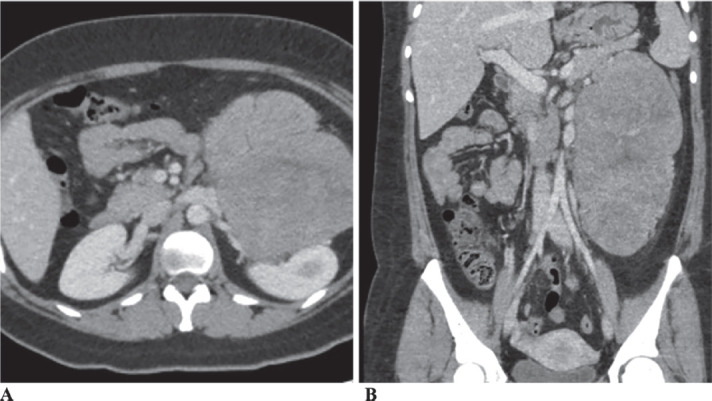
CT Abdomen (1A-Axial section and 1B-Coronal section) showing a 21 × 15 cm mass abutting the left kidney, left renal vessels, distal pancreas and spleen.
